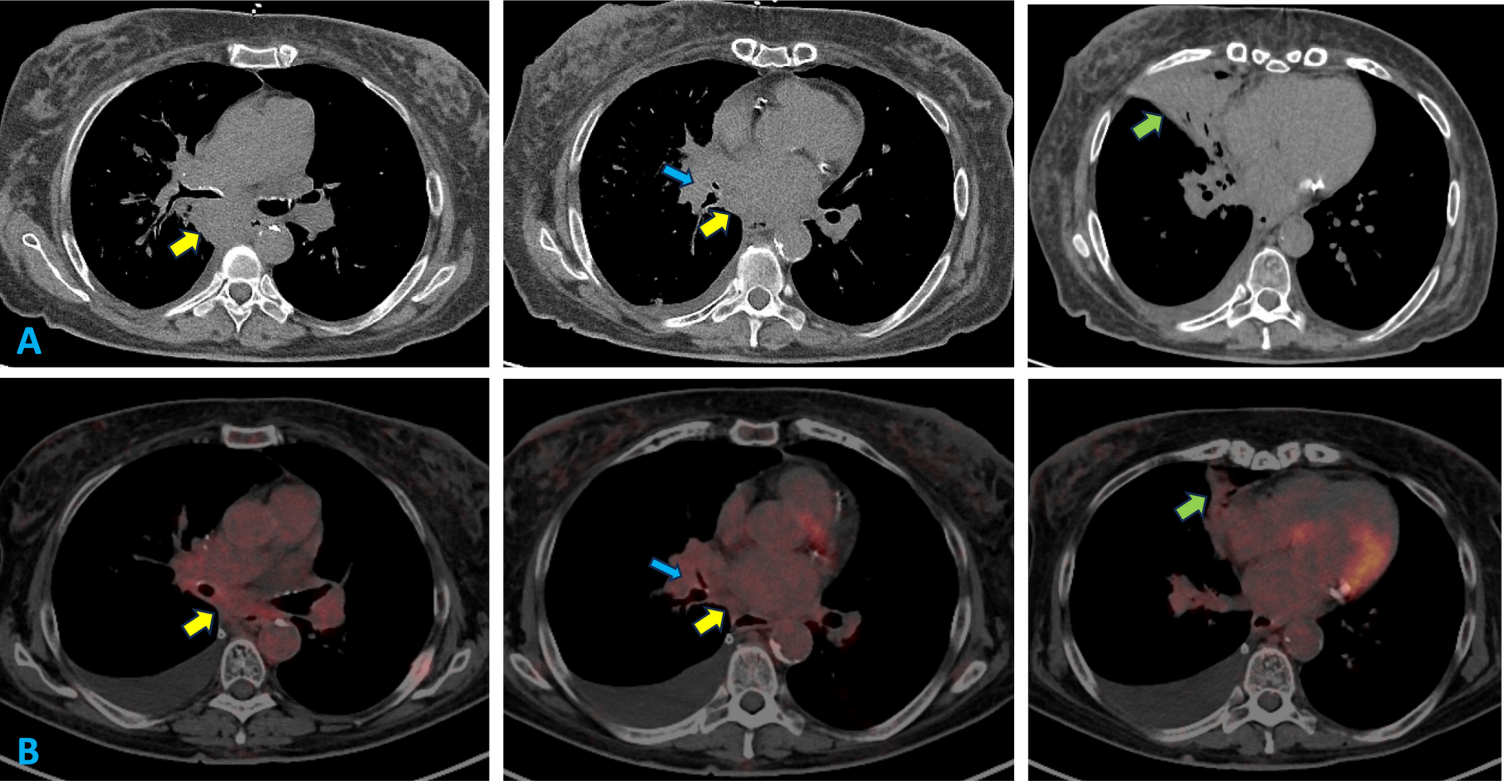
Upper panel (A) Computed tomography images showing enlarged subcarinal (yellow arrows) and right hilar (blue arrow) nodes, causing narrowing of the right middle lobe bronchus and collapse of the right middle lobe (green arrow). Lower panel (B) Positron Emission Tomography images showing a decrease in size of subcarinal (yellow arrows) and right hilar (blue arrow) nodes, and improvement in the collapse of the right middle lobe (green arrow).
